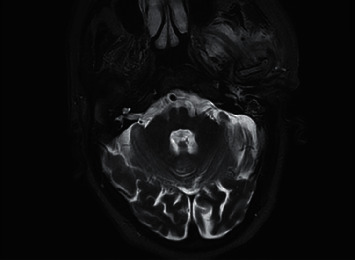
T2-weighted axial MRI prior to treatment being commenced for NOE again demonstrating inflammatory changes centred around the left temporomandibular joint.
